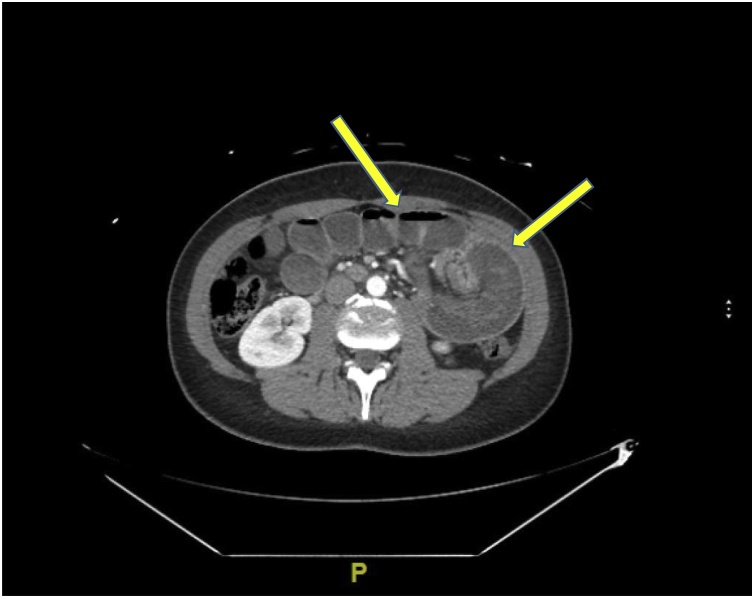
Axial CT scan of abdomen/pelvis showing small bowel obstruction with dilated loops of small bowel. Seen is a large mass in the left upper quadrant which is a closed loop bowel obstruction at the anastomotic site - J-J anastomosis. Swirling (volvulizing) of the mesentery is also seen.
